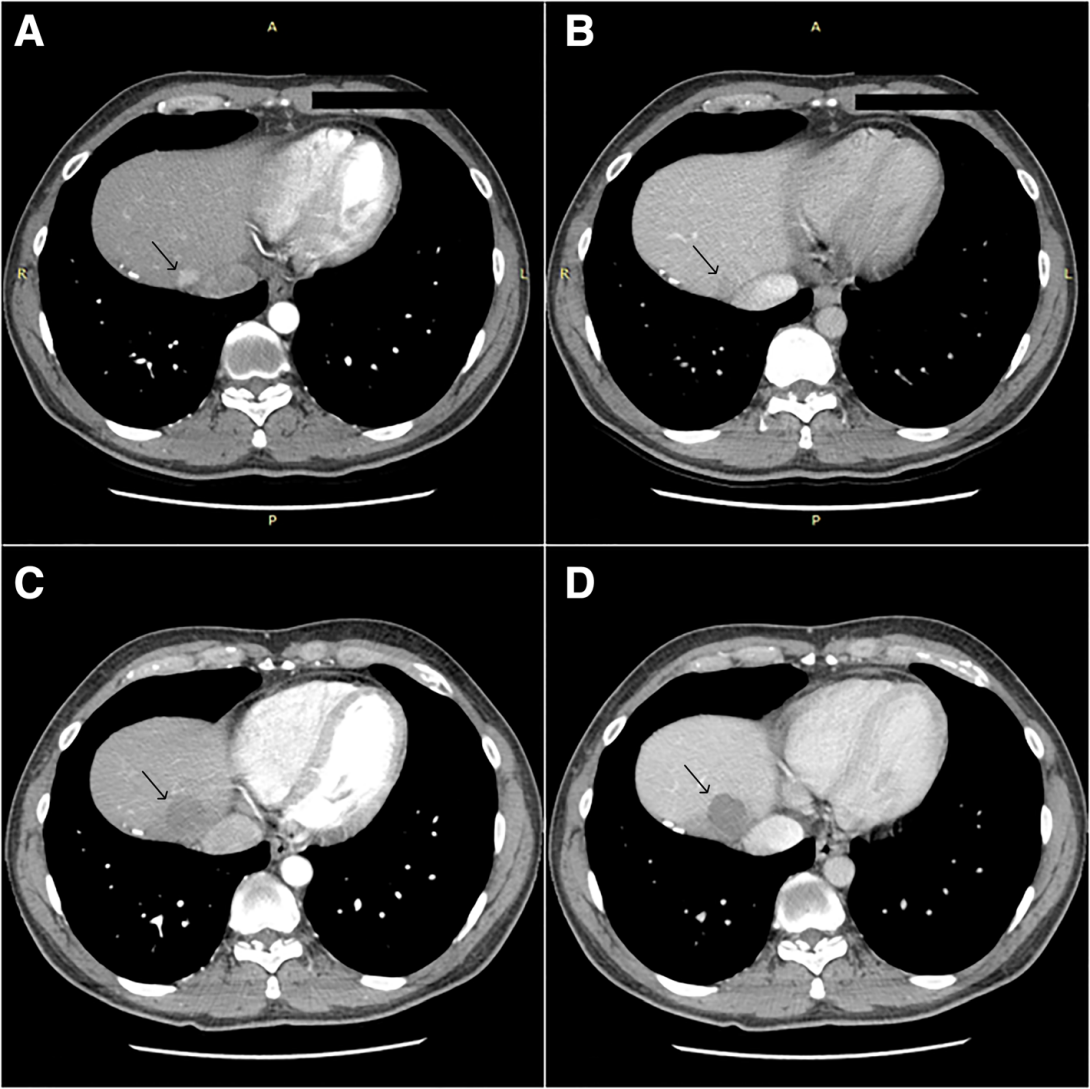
a and b Computed tomography abdominal scan, hepatocellular carcinoma nodule of 18 × 14 mm near the confluence of the middle hepatic vein and the inferior vena cava (arterial and venous phase). c and d Three-month follow-up computed tomography abdominal scan, complete necrosis of the nodule (arterial and venous phase). The area of the nodule pre-procedure and postprocedure is indicated by an *arrow*
